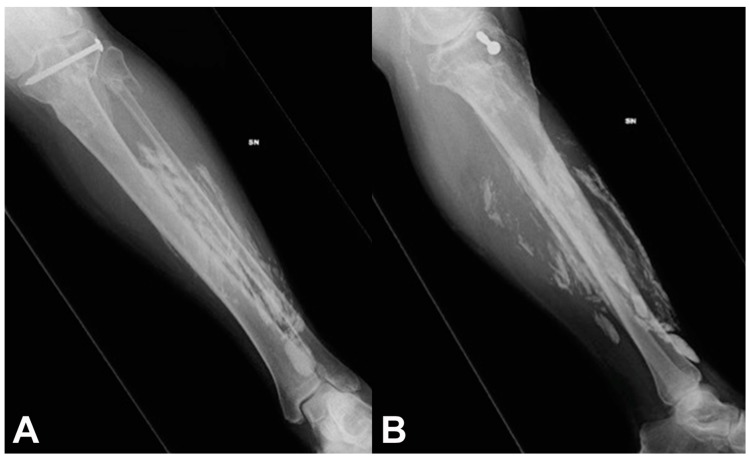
(A) Anteroposterior and (B) lateral radiographs of the left leg of a 68-year-old man with biopsy diagnosed calcific myonecrosis of the leg show extensive calcifications at the soft-tissue of the anterior and posterior leg.
